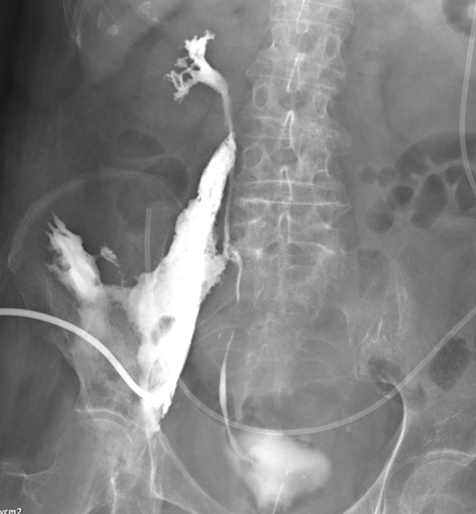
X-ray fluoroscopy. When a contrast medium was injected through the IPA drain, the kidneys, ureter, and bladder were also contrasted.
